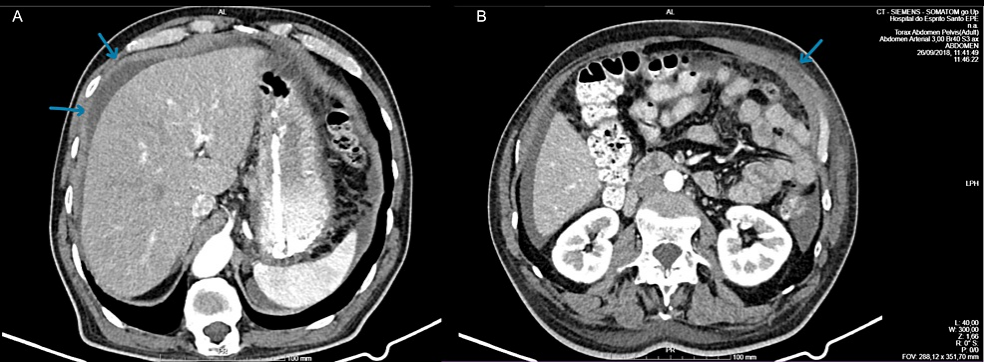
Thoraco-abdominal-pelvic CT performed in 2018 showing moderate ascites with mesenteric densification CT: computed tomography
